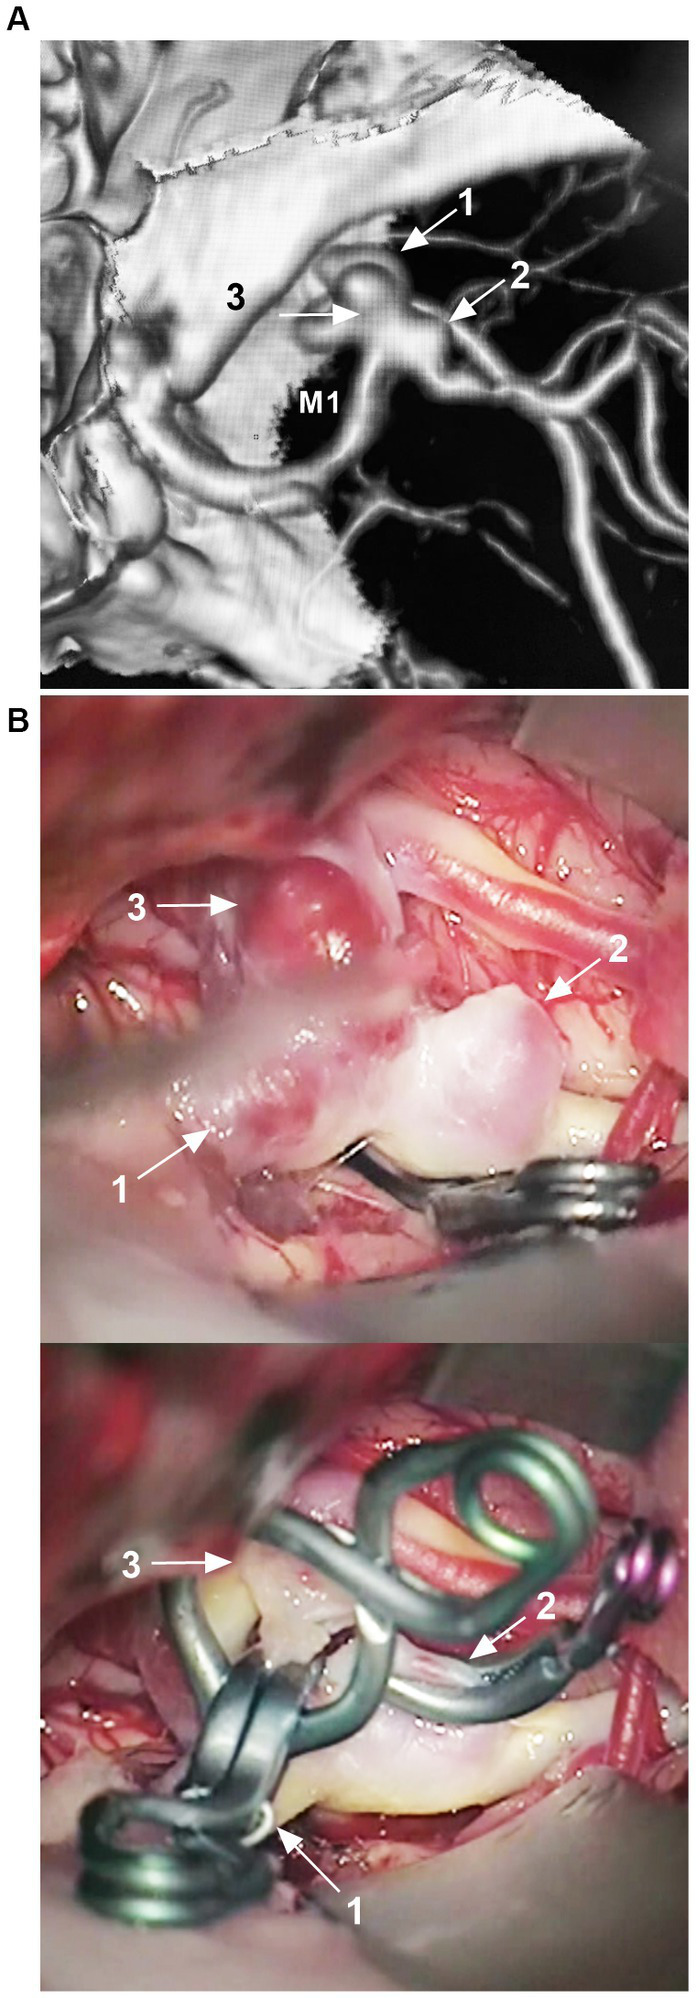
Clipping of MCA aneurysm. (A) CTA showing a right MCA bifurcation aneurysm, with three blebs (arrows 1, 2, and 3). (B) Upper panel: Intraoperative CTA image showing the aneurysm with three blebs (arrows 1, 2, and 3), MCA trunk had been clipped temporarily; Lower panel: Intraoperative image showing three blebs (arrows 1, 2, and 3) of the aneurysm that was clipped. CTA, computed tomography angiography; MCA, middle cerebellar artery; M1, first segment of the MCA.
